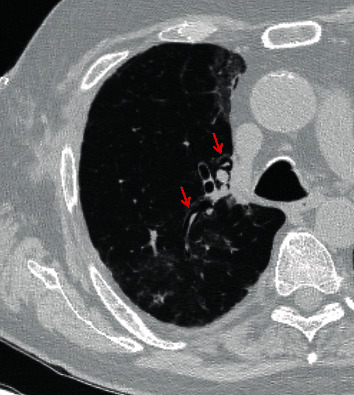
Initial axial chest CT two weeks prior shows interstitial emphysema along the bronchovascular bundle (red arrows).
